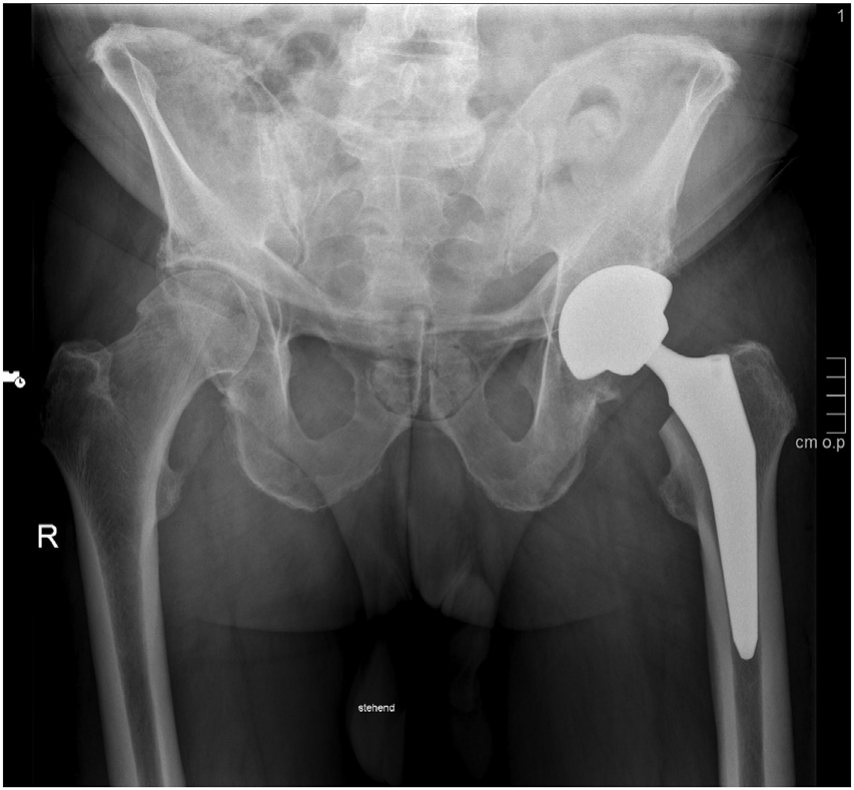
Postoperative radiograph obtained after the procedure.
